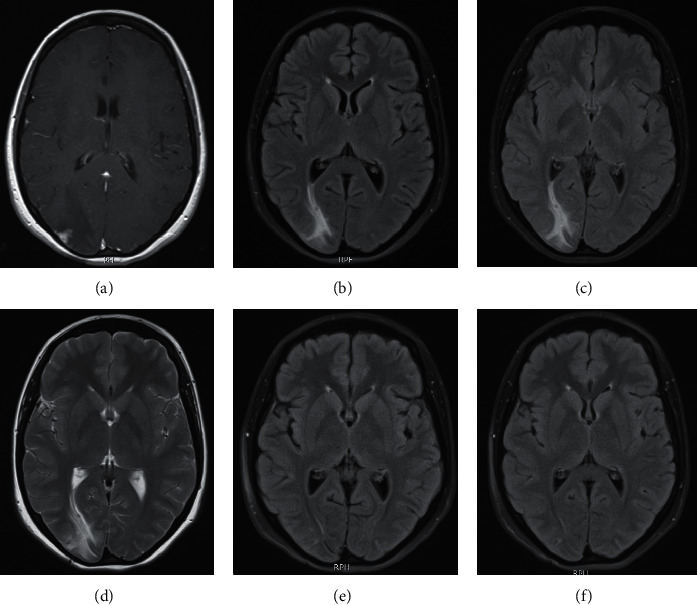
Baseline and evolution of cerebral MRI in neurobrucellosis patient. (a) Initial presentation. (b) 1 month later. (c) 2 months later. (d) 7 months later. (e) 6 months after treatment. (f) 1 year after treatment.
